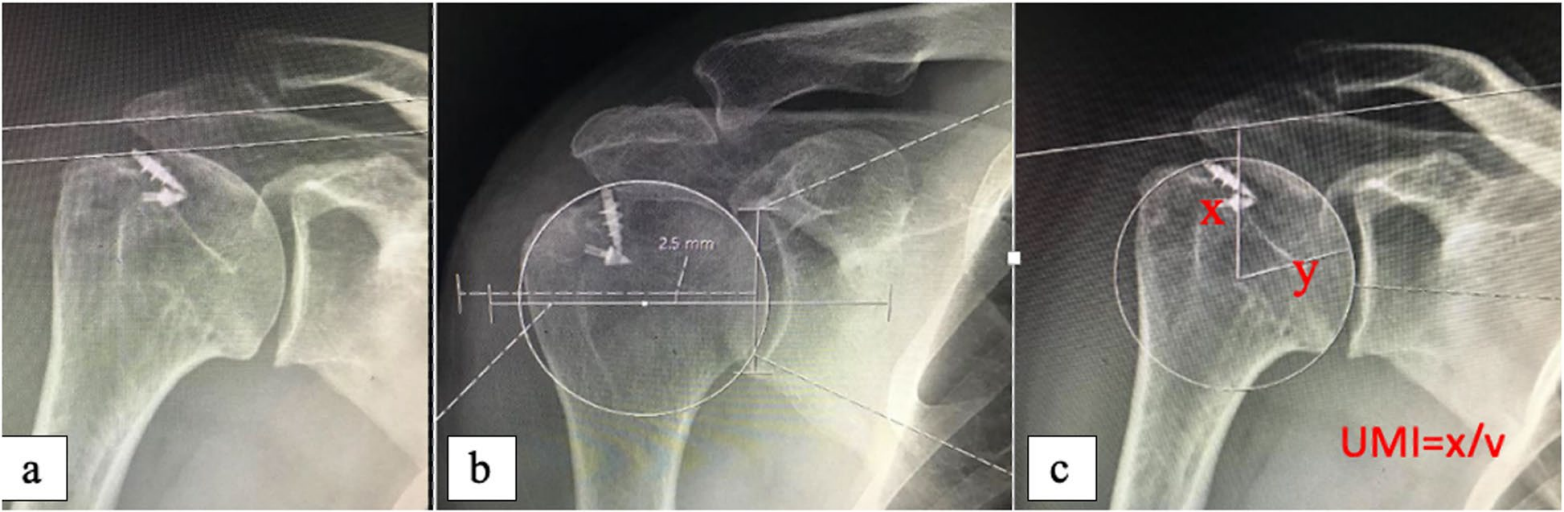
Radiological measurements at X-ray. a Acromiohumeral distance measurements. b Humeral migration measurements, c Upper migration index formula and measurements
